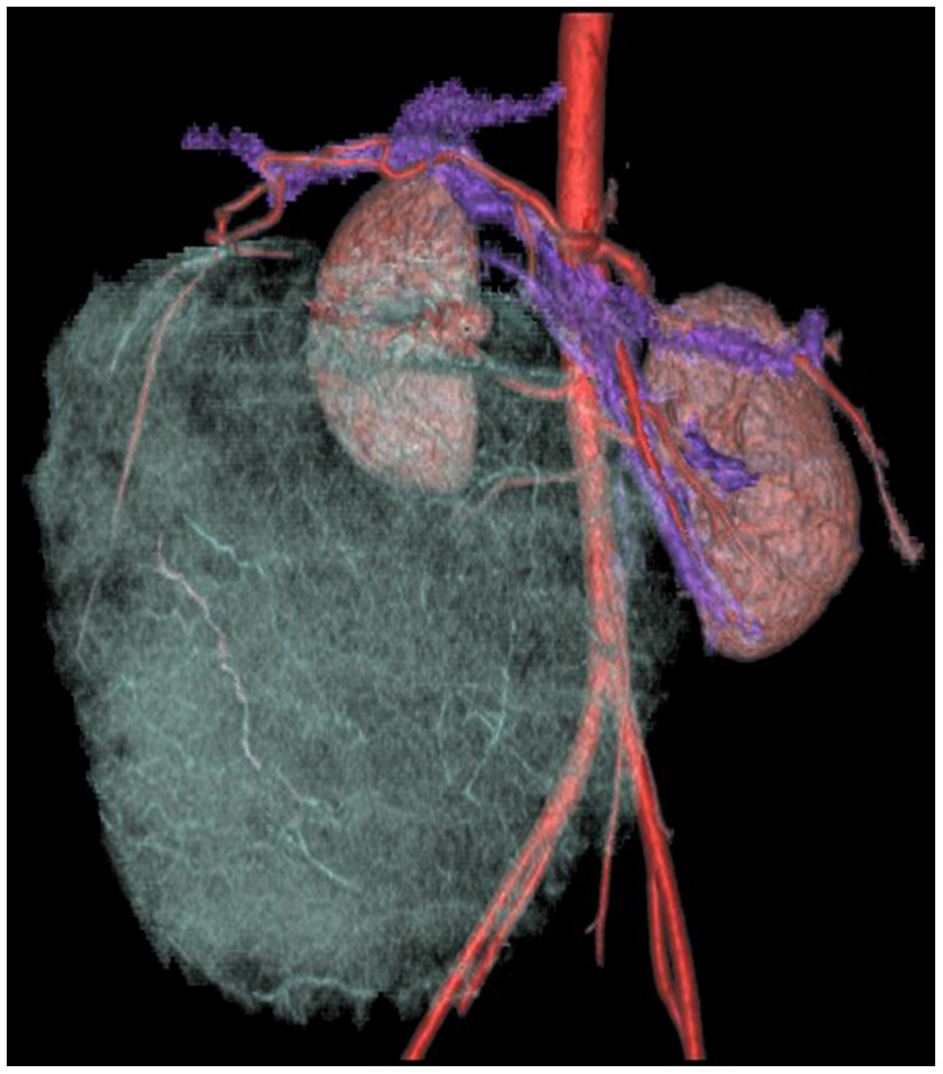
Enhanced CT of progressive enhancement of the solid part of the lesion in the portal venous phase, the lesion closely related to the liver, and the abdominopelvic fluid accumulation.
